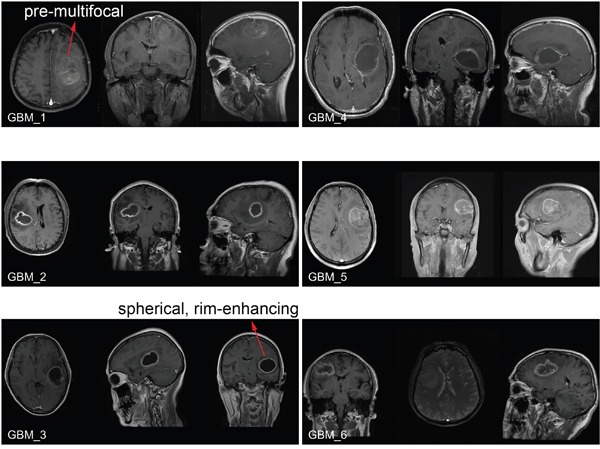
Brain MRI images of GBM patients enrolled in the clinical cohort Before surgery, the tumor growth was examined by the T1 post-contrast MRI imaging. The pre-multifocal growth of MBD3-low GBM features a rapid tumor expansion, aggressive infiltration to the surrounding normal tissue, and poor survival. In comparison, the spherical, rim-enhancing MBD3-high GBM features a relatively mild growth, confined edge, and better survival.
